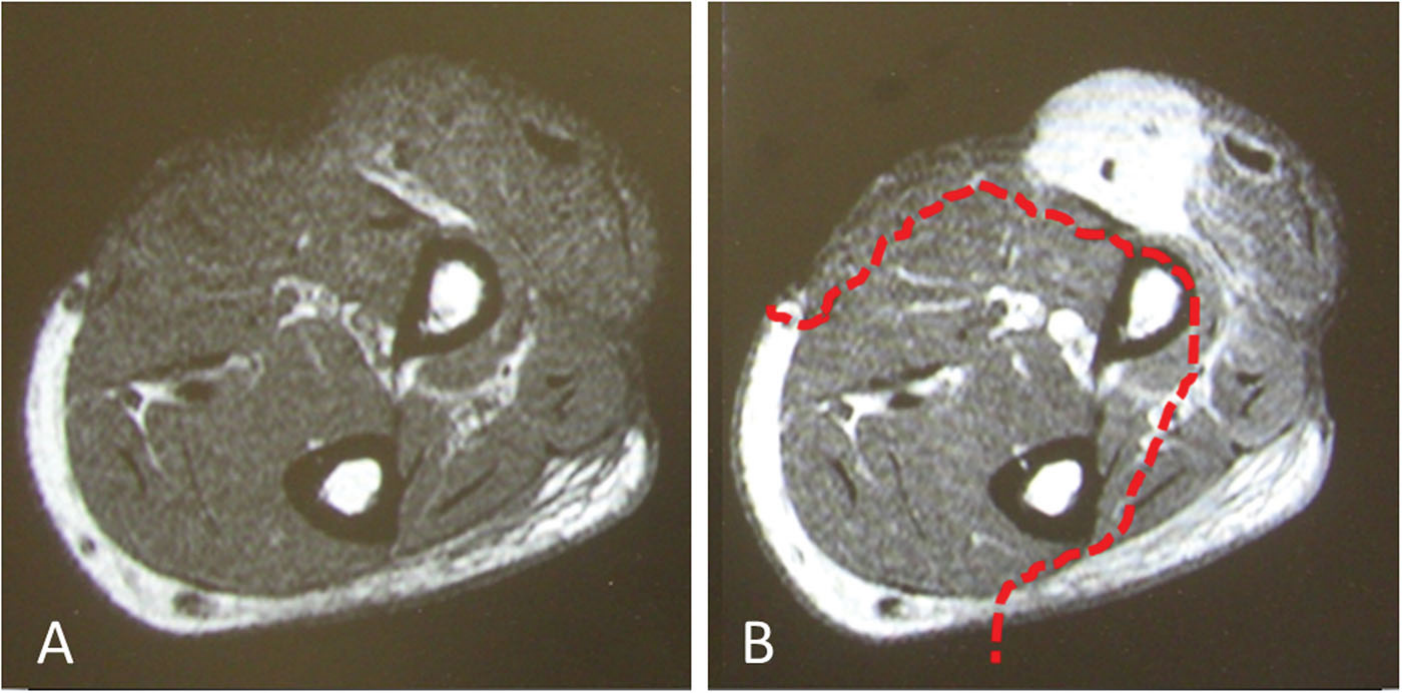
Images prior to reoperation. (A) MRI T1 weighted image. (B) MRI T1 weighted image with gadolinium enhancement. The dotted line indicates the extent of tumor resection. MRI: Magnetic resonance imaging.
